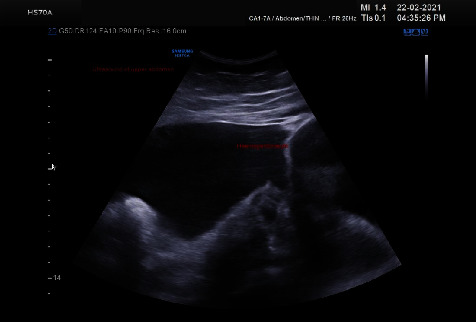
Ultrasound of the upper abdomen showing massive hemoperitoneum.
